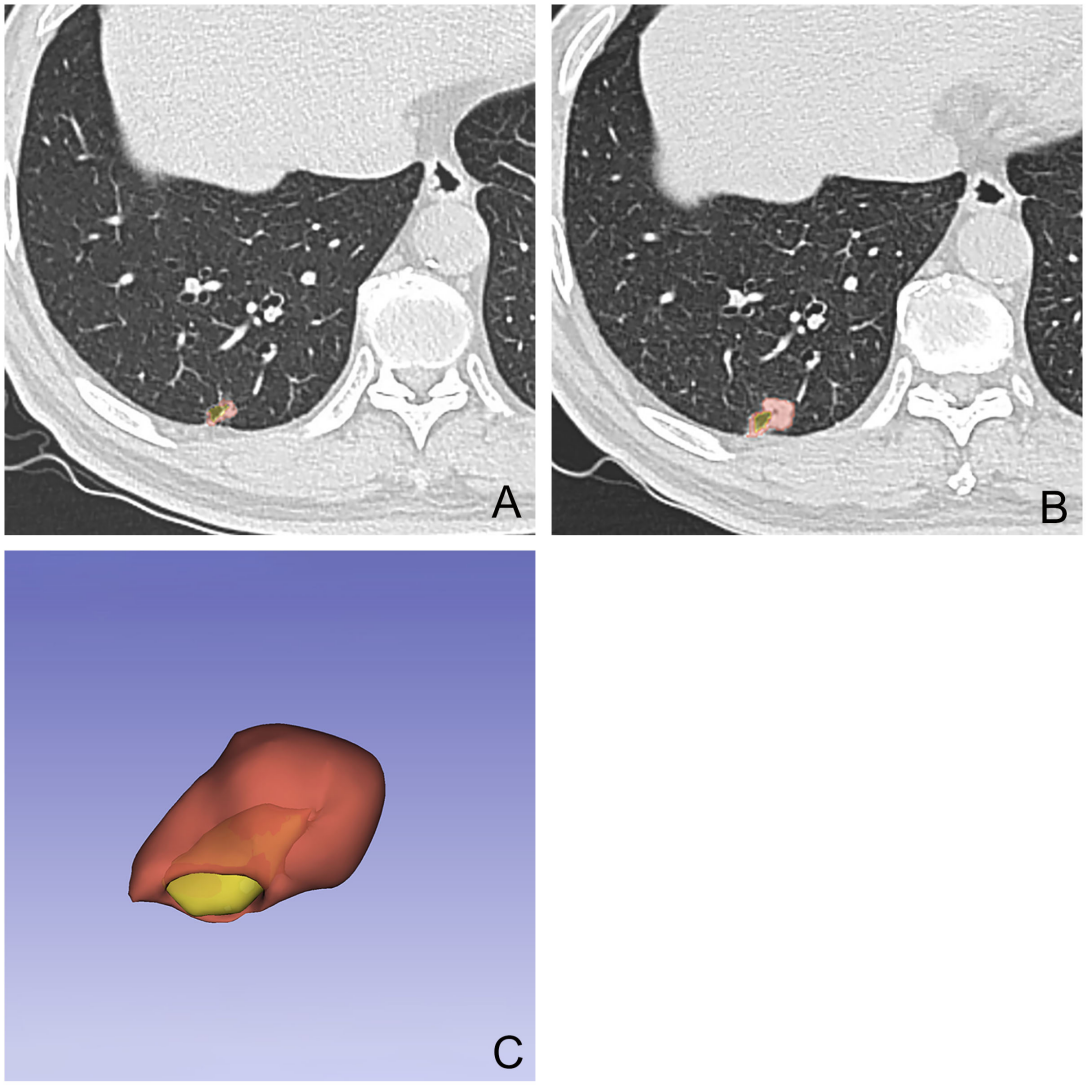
A 54-year-old male patient with a nodule with cystic airspaces identified during initial examination (A). Follow-up CT at 12 months showed increased nodule size (B). The final examination’s 3D tumor model displays the cystic area in yellow and the tumor in red (C).
